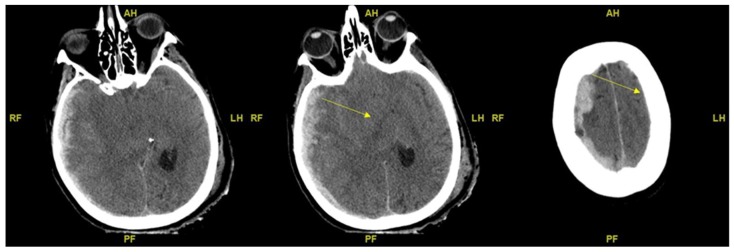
The axial CT scans evidence diffuse brain edema and swelling, with severe midline shift due to multiple brain contusions and acute subdural and subarachnoid hemorrhage. Cortical sulci, Sylvian fissure, third ventricle, and perimesencephalic cistern are either compressed or not visible. From left to right side yellow arrows show the third ventricle compressed and dislocated and the cortical sulci compressed and pushed toward the skull.
